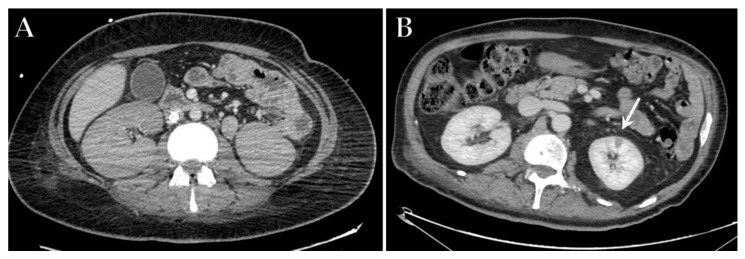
Axial contrast-enhanced CT image of an ICU patient with COVID-19 ARDS in ECMO treatment that developed kidney injury, showing enlarged kidney with a loss of corticomedullary differentiation (A). Axial contrast-enhanced CT image of a different patient with severe COVID-19 admitted to ICU demonstrating a wedge-shaped region of hypoattenuation in the left kidney (white arrow) consistent with renal infarction (B).
